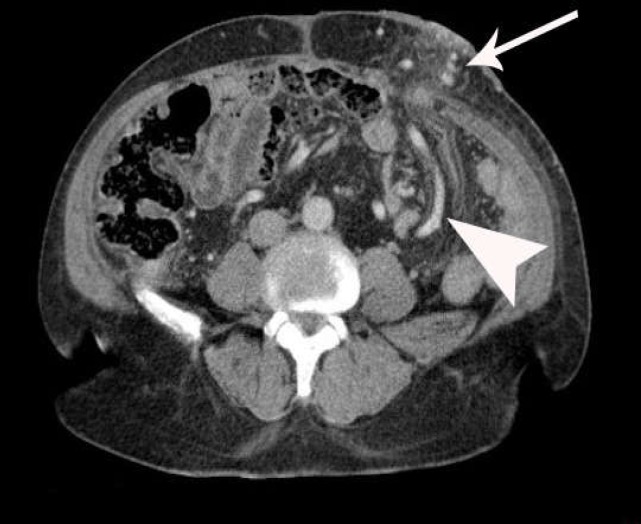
52 YO male with UC, PSC& colostomy due to colon cancer who presented with massive stomal bleeding. Arterio-portal phase CT SCAN shows parastomal varices (arrow) and enlarged mesenteric afferent tributary (arrowhead)
